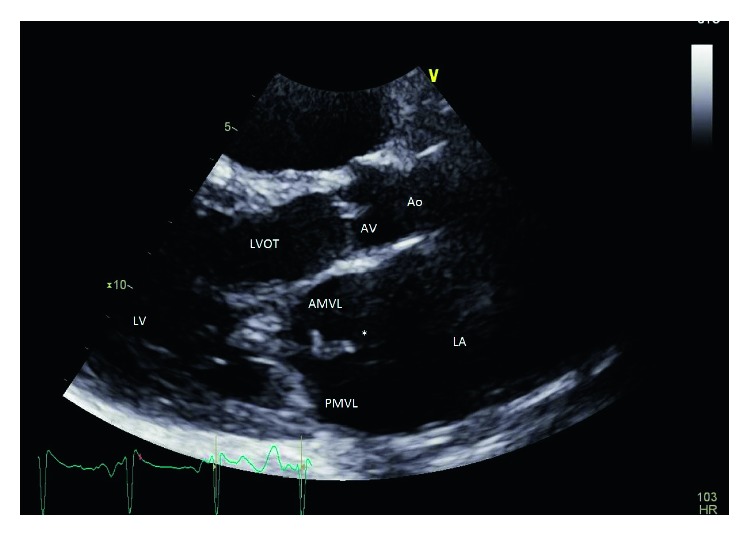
Transthoracic echocardiogram in parasternal long-axis view. Vegetation emanating from the anterior mitral valve leaflet is marked by an asterisk. LA: left atrium; LV: left ventricle; LVOT: left ventricular outflow tract; AV: aortic valve; Ao: aorta; AMVL: anterior mitral valve leaflet; PMVL: posterior mitral valve leaflet.
